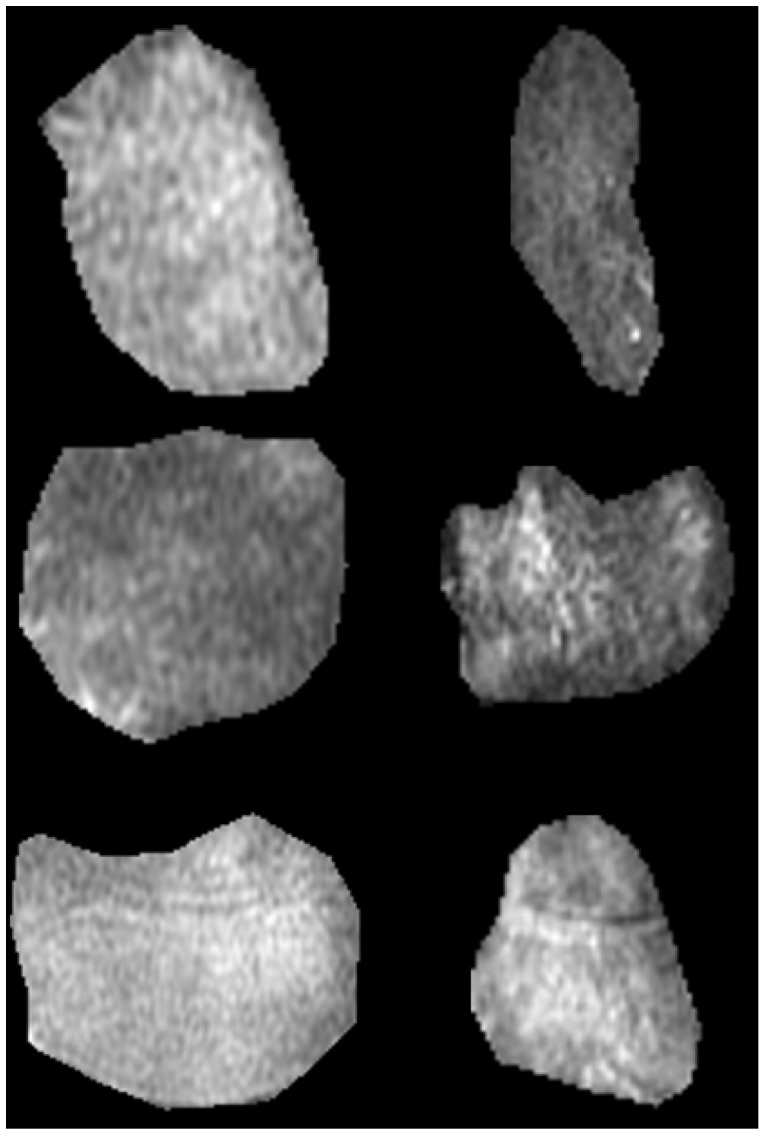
Samples of region of interest for the three categories and two MRI sequences; bone marrow edema (first row), injured (middle row), and osteoarthritis (last row); PD-FSE (left column) and STIR (right column).
